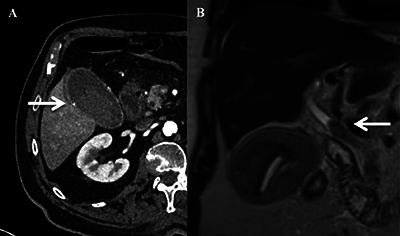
(A) Axial CT angiography confirming the diagnosis of cystic artery pseudoaneurysm (arrow). (B) Coronal T2-weighted MRI image showing an obstructing clot in the common bile duct (arrow).
